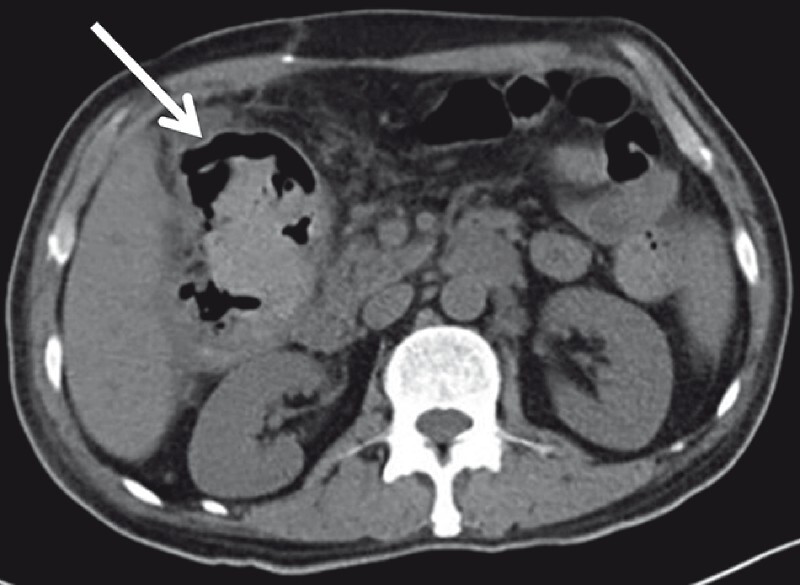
A CT taken 7 days post-operation, revealing partial improvement in the colonic obstruction and decreased density in the colon proper.
